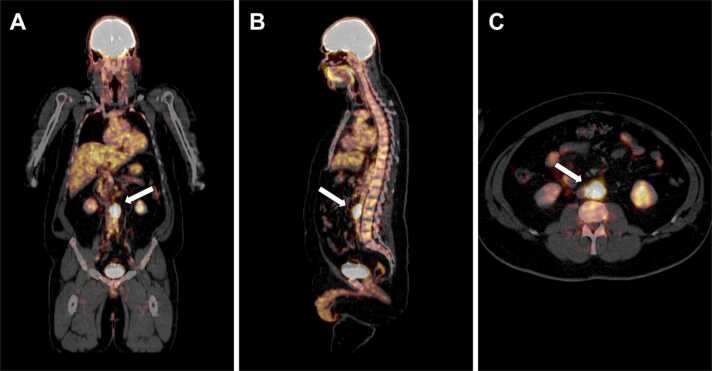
A 48-year-old patient who was admitted to the emergency department with persistent flank and back pain in the lumbar spine area. In an initial CT scan of the lumbar spine, an unknown tissue formation was accidentally discovered which was located around the infrarenal abdominal aorta. A clear tracer uptake *(white arrows)* has been observed in a subsequent PET/CT examination, finally establishing the diagnosis of large-vessel vasculitis.
